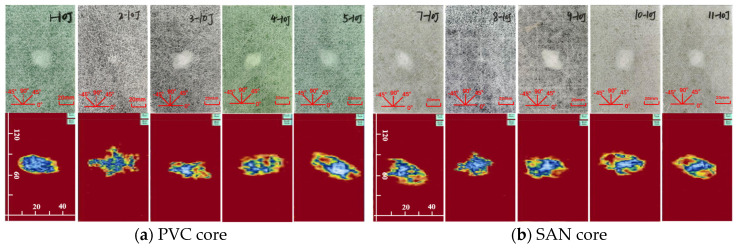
Physical picture of 10 J impact damage and ultrasonic C-scan image.
